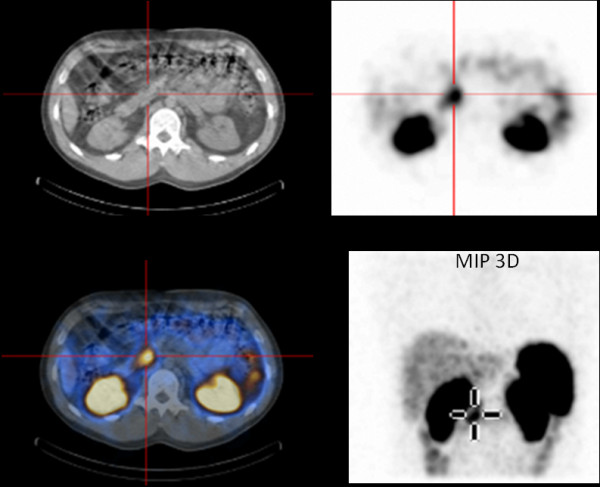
Somatostatin receptor scintigraphy (OctreoScan®) of the patient: single-photon emission computed tomography was performed 3 hours after injection of 200MBq^111^In-pentetreotide and showed a focal uptake at the junction between the head and the body of the pancreas. MIP 3D; Maximum intensity projection, three dimensional.
